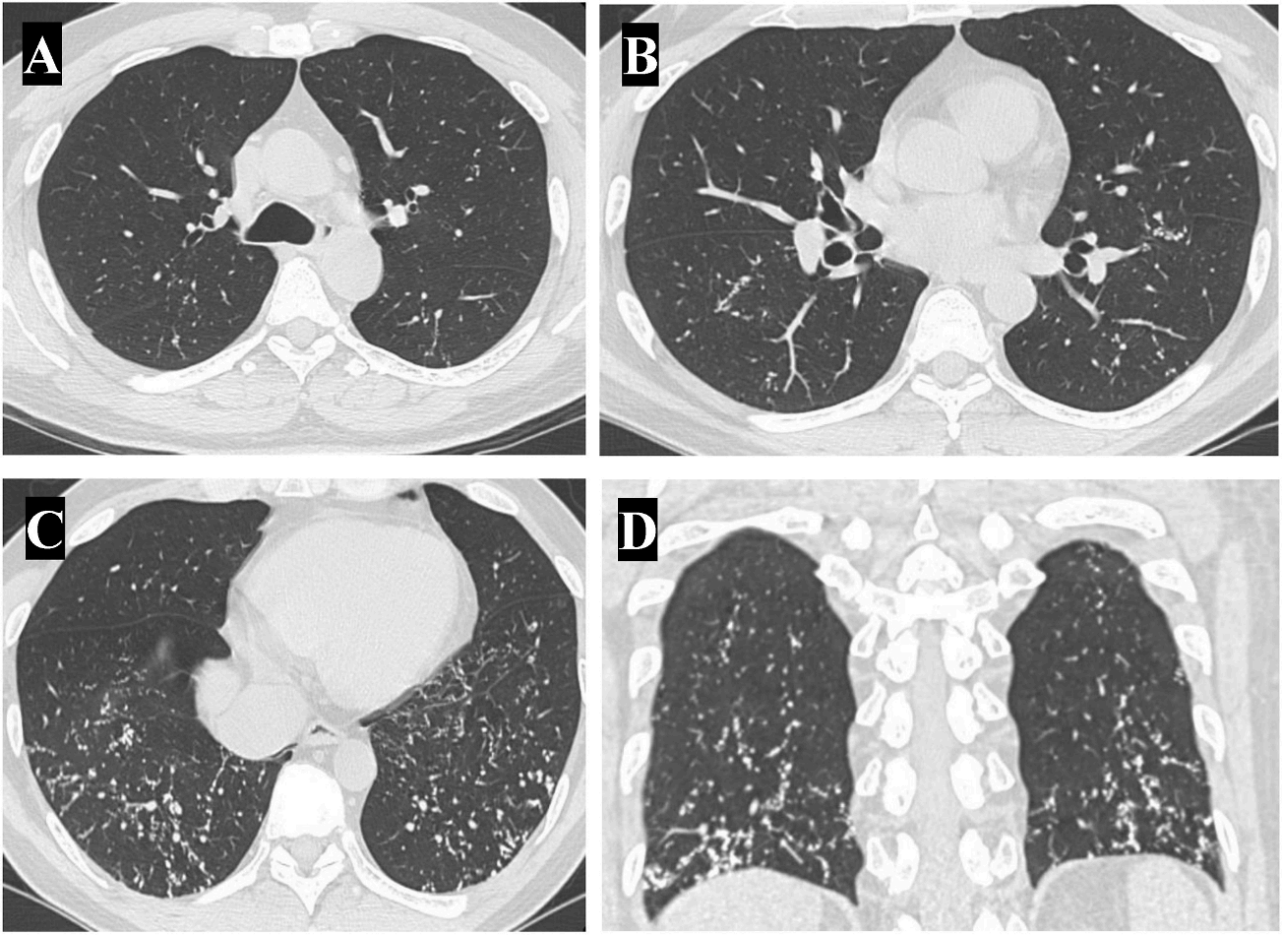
Three different computed tomography axial cuts (A–C), one coronal cut (D). Posterior basilar selective micronodular disease consistent with pulmonary ossification.
